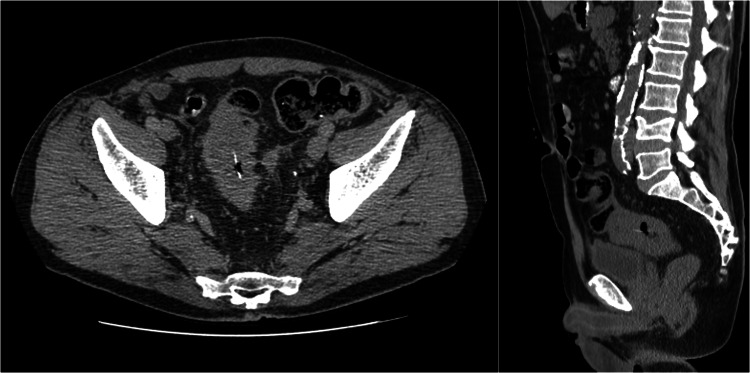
Abdomen and pelvic CT scan revealed a linear calcic foreign body at the distal sigmoid, with apparent wall thickening and a possible perforation.
